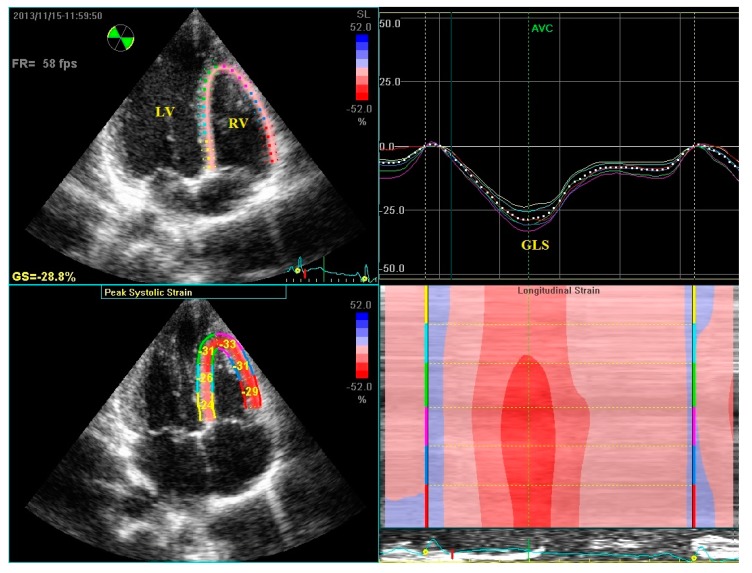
Example of global systolic strain (GLS) in the right ventricle from apical four chamber view. RV: right ventricle, LV: left ventricle.
